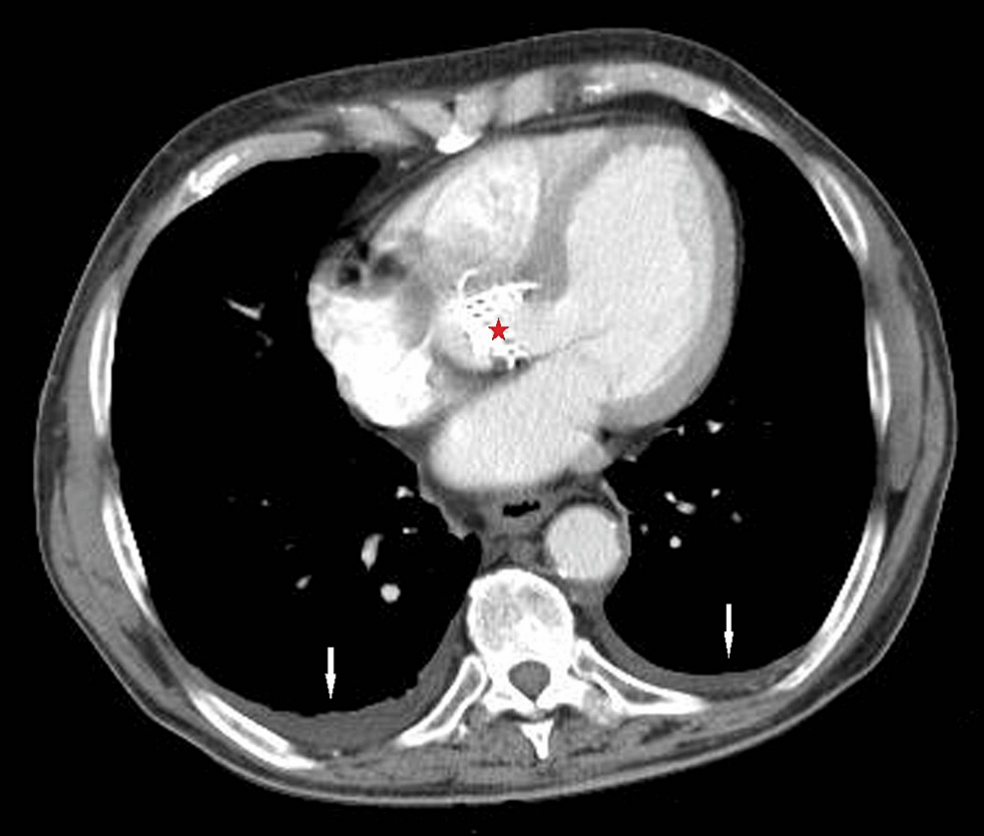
Chest CT scan, where the bioprosthetic aortic valve is visible (red asterisk), showing bilateral pleural effusions (white arrows).
